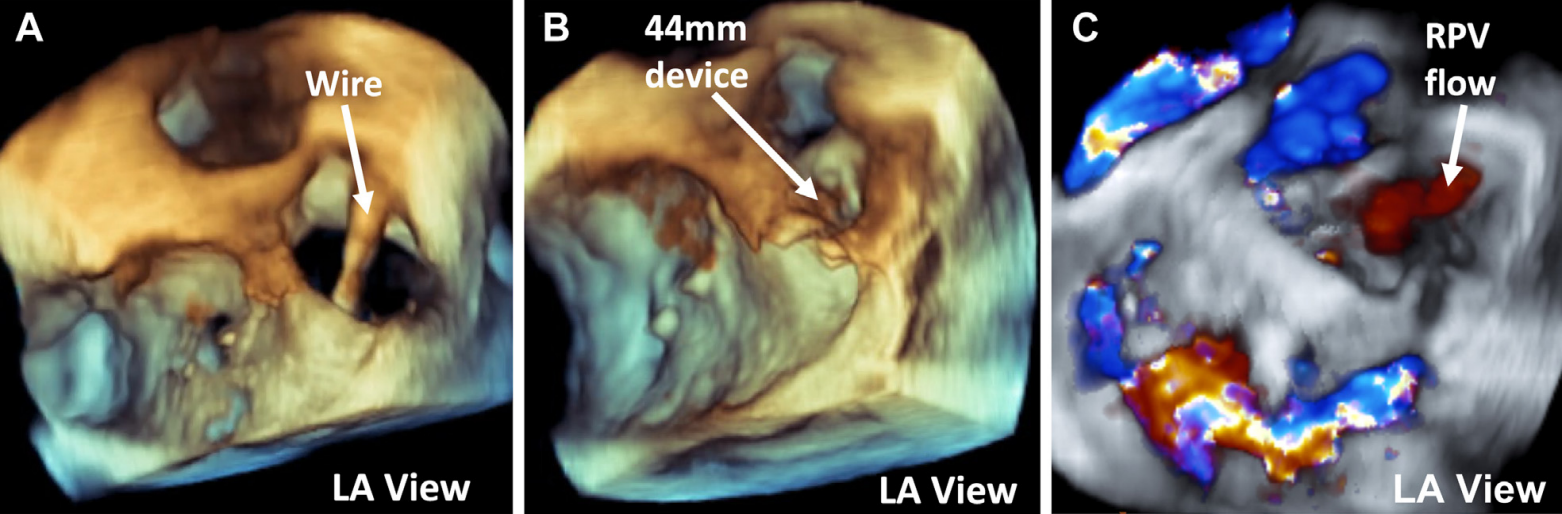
Procedural 3D Transesophageal Echocardiogram (A) 3D TEE of wire through defect from left atrial (LA) view. (B) 3D TEE with color Doppler of 44-mm device deployed into defect from LA view. (C) 3D TEE with color Doppler of 44-mm device deployed into defect without obstruction of the right pulmonary venous (RPV) flow.
